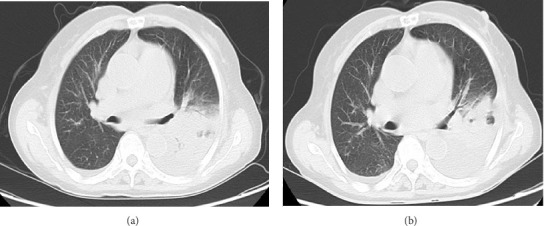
The changes of pulmonary window on CT: (a) and (b) were pulmonary inflammatory lesions at admission and 1 week after treatment. The pulmonary inflammation was more absorbed, accompanied by pleural and pericardial effusion after treatment.
